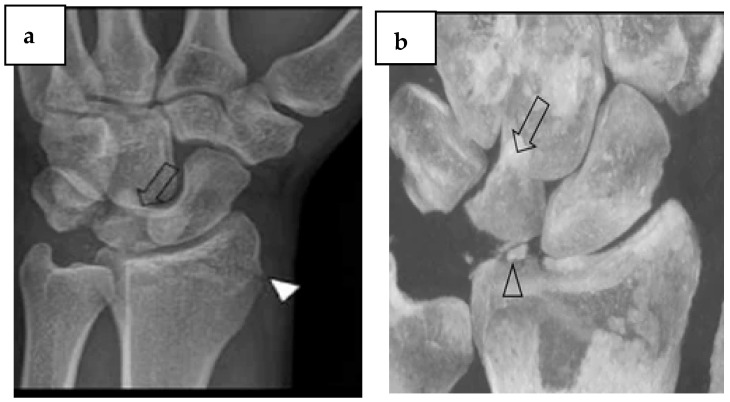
Complex fracture involving multiple intra-articular fracture fragments, perilunate dislocation, and involvement of the dorsal side of the radius’s styloid process. (a) The distal radius fracture (arrowhead) and perilunate dislocation (arrow) are visible in the CR (oblique view). (b) 3D reconstruction of CBCT images helpful in assessing the carpal bone displacement (open arrow) and the presence of further fracture fragments (open arrowhead). Published with permission from [18]. Copyright 2018, Springer Nature.
